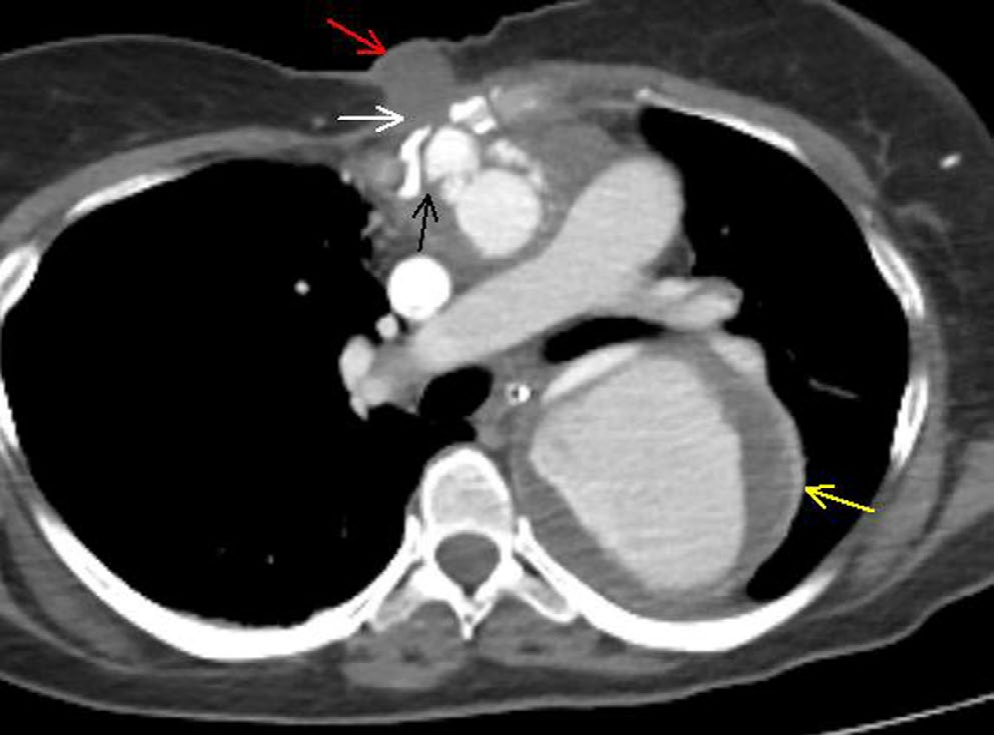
Chest CT angiography showing the aorto‐cutaneous fistula (red arrow). Note the sternal dehiscence (white arrow), the pseudoaneurysm, and surrounding hematoma between the prosthetic ascending aorta and the back of the sternum (black arrow) along with the aneurysmatical dilatation of the false lumen of the thoracic aorta after the prosthetic replacement (yellow arrow), which were unaltered compared to follow‐up CTs. There was no evidence of free thoracic aorta rupture
